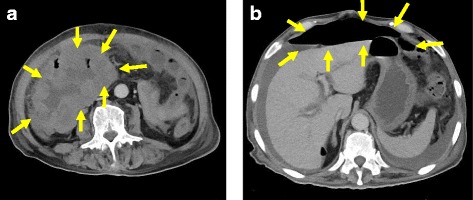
a Image of a small intestinal tumor in our patient. The arrows indicate a small intestinal tumor. b Image of contrast-enhanced computed tomography (CT) in our patient. The arrows indicate free air
